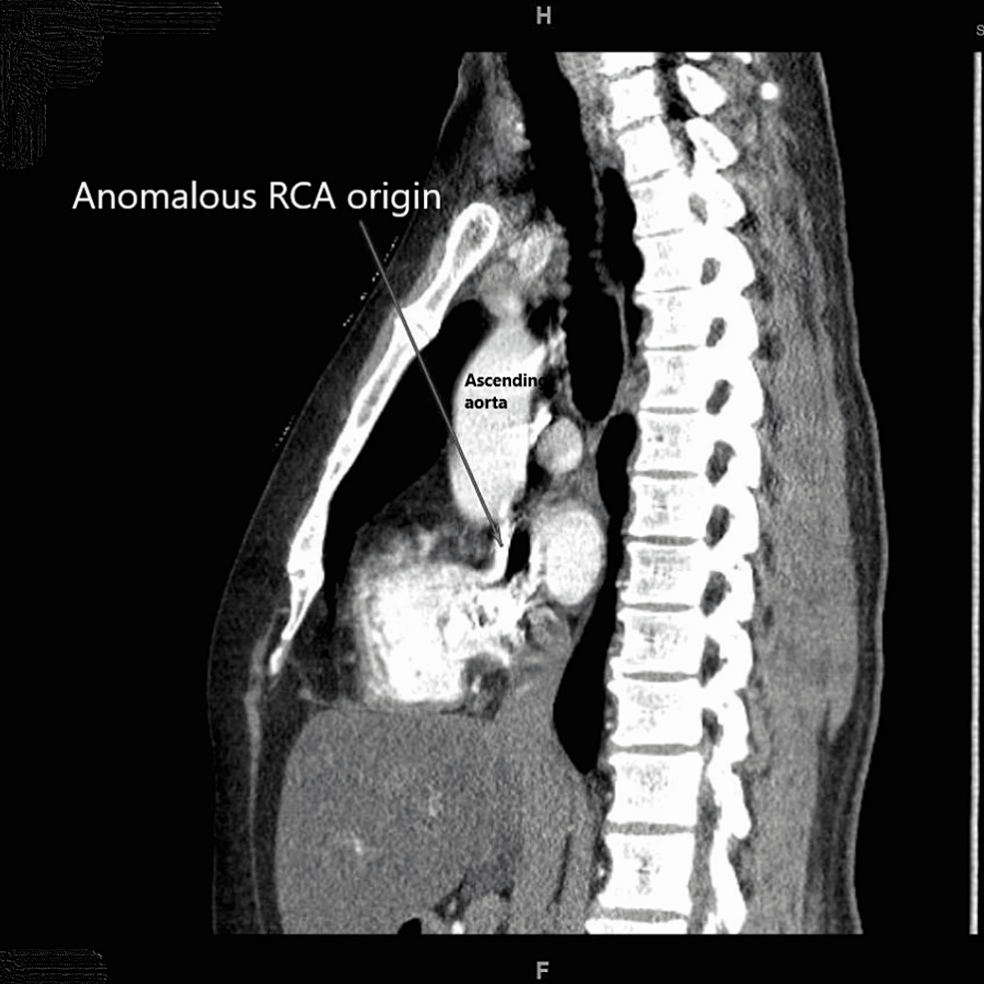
CTA chest shows an incidental anomalous origin of the right coronary artery from the left sinus of Valsalva (sagittal view).
